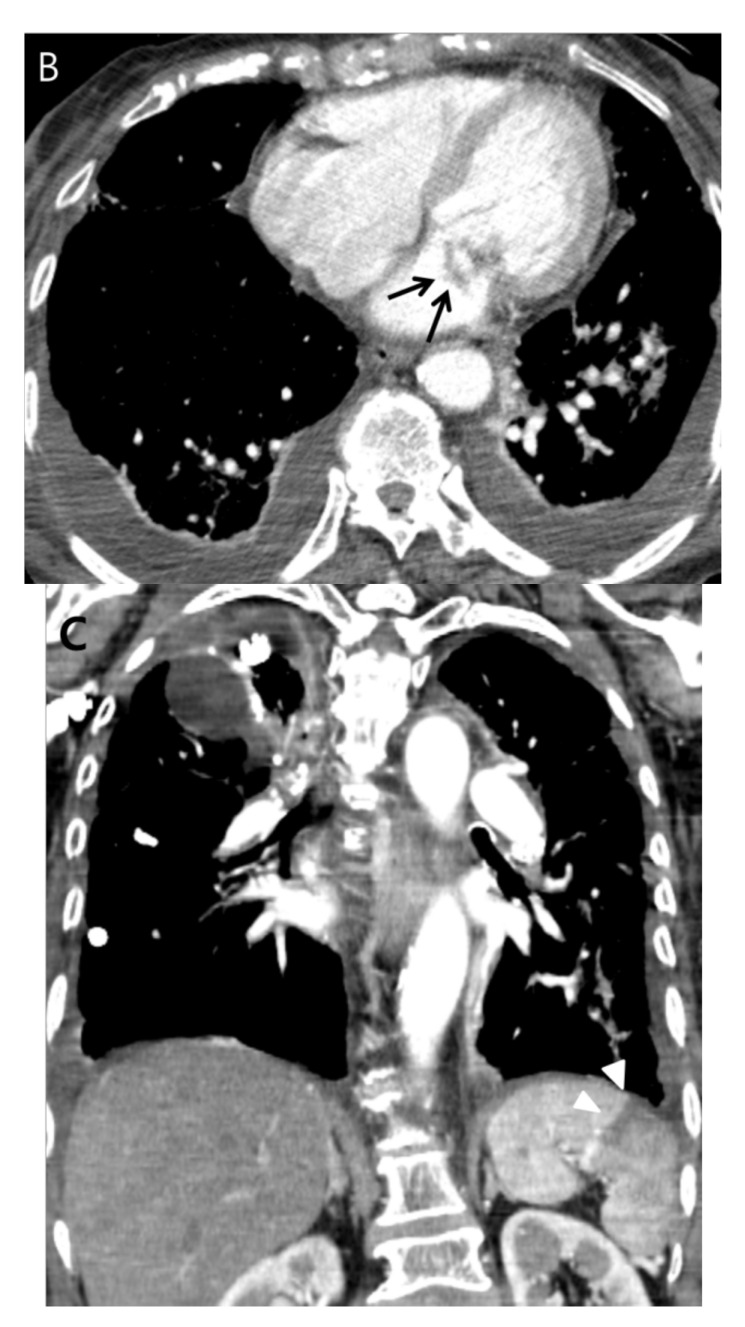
A case of an indirect-oscillation sign with splenic infarction on CT. IE involves the mitral valve on routine chest CT in a 75-year-old female patient. (A) Note the interlobular septal thickening and ground glass opacities in both lungs, suggesting the presence of pulmonary edema on a coronal CT image. (B) On an axial CT image, elongated nodular thickening with a blind end (double arrows), an abnormal orientation from the normal mitral valve, and attachment to the mitral valve (i.e., indirect-oscillation sign) may indicate the presence of vegetation (arrows). (C) Note the splenic infarction on a coronal CT image (arrowheads).
